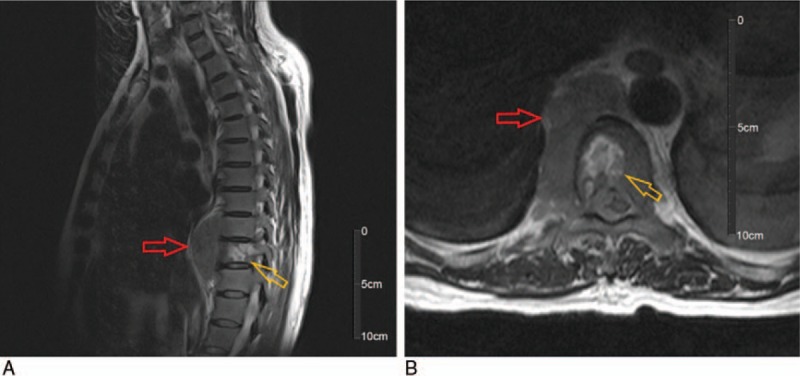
Magnetic resonance imaging (T2-weighted) revealed lesion near centrums 9–11 (red arrow) that invaded the vertebral body (yellow arrow). (A) sagittal and (B) transversal images.
